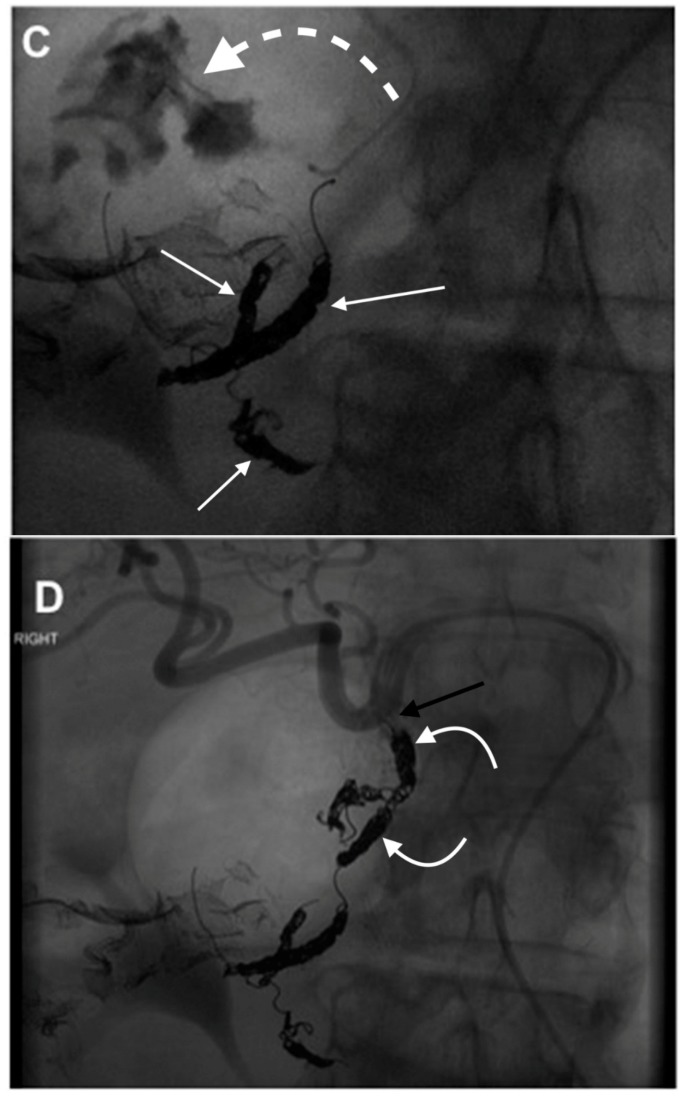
This 78 year old woman presented with melena, hematemesis, hypotension, and acute blood loss anemia. An endoscopy was not performed, given the high volume of blood which was felt to likely obscure the (A) Computerized Tomography (CT) images, demonstrating the duodenal perforation (white arrow) in the posterior wall with abnormal appearing GDA coursing directly adjacent to the perforation (white arrowhead). The (B) celiac angiogram shows the origin of the GDA (curved black arrow). The (C) branches of the GDA that were distal to the duodenal ulcer were coil emobolized (white straight arrows) and the repeat angiogram from the proximal to mid GDA, showed a contrast extravasation into the duodenum (curved dashed arrow). (D) Coil embolization (curved white arrows) was done across and proximal to the site of the extravasation up to the origin of the GDA from the common hepatic artery (black arrow).
